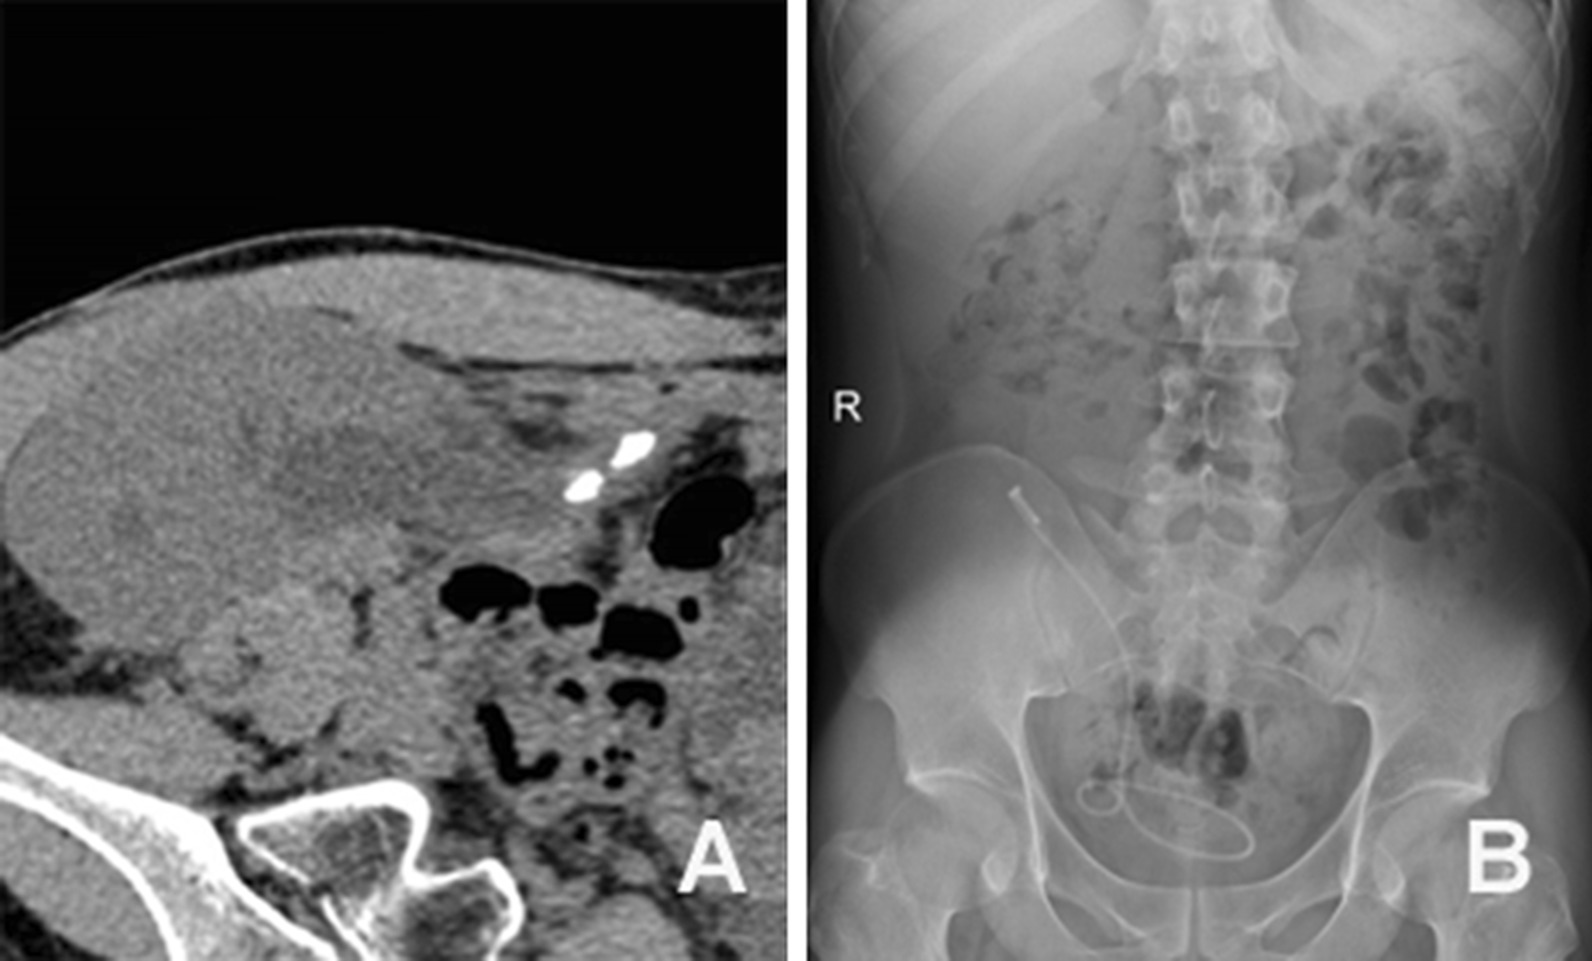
The placement of a double-J stent in a 39-year-old Chinese male developing ureterovesical junction stenosis and kidney calculi. a The computed tomography revealed multiple allograft stones. b The KUB film revealed that the double-J stent was inserted into the pelvis of the renal allograft
